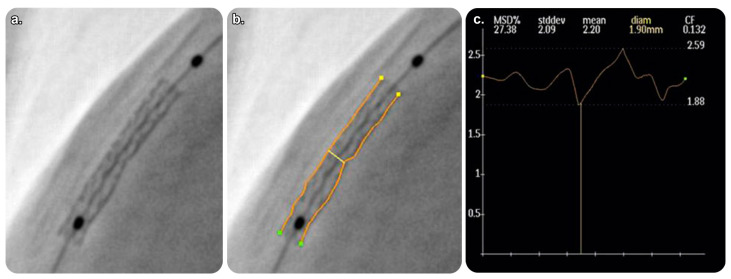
Quantitative analysis of StentBoost software images. (a) Enlarged and enhanced StentBoost image. (b) Manual tracing of longitudinal stent edges to avoid inadequate stent expansion. (c) Minimum stent diameter determined automatically. Reprinted from “Assessment of optimum stent deployment by stent boost imaging: Comparison with intravascular ultrasound” by Tanaka et al. [33] with written approval from the corresponding author and publisher (Heart and Vessels; Copyright 2013).
